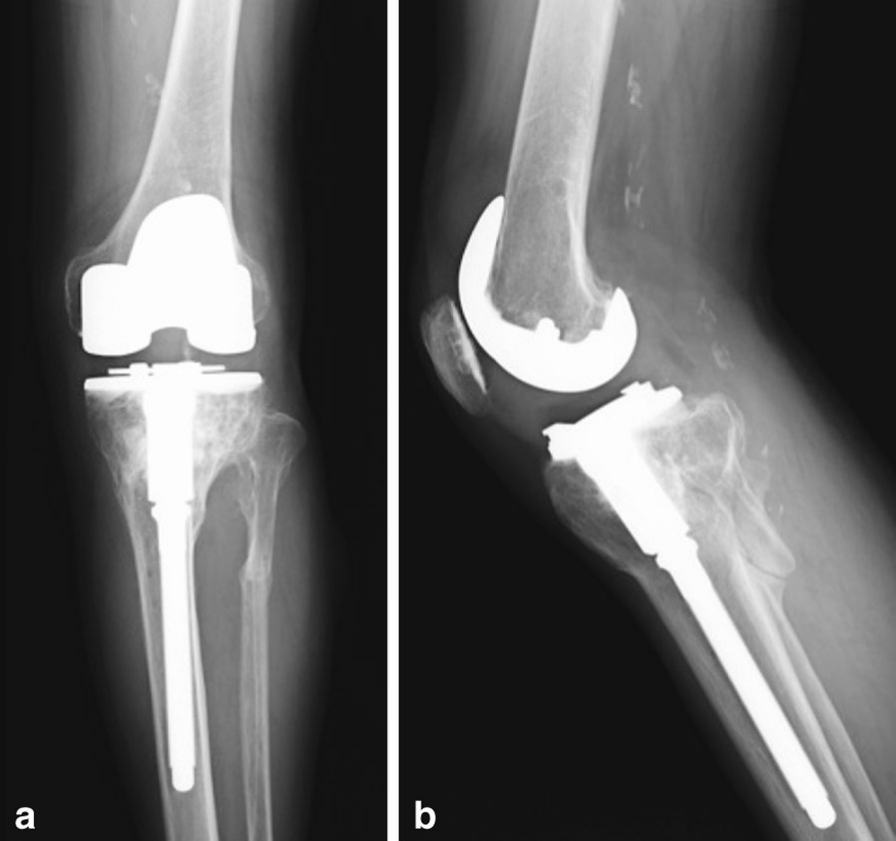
Radiographs at 24 months postoperatively. a Anteroposterior view. b Lateral view
